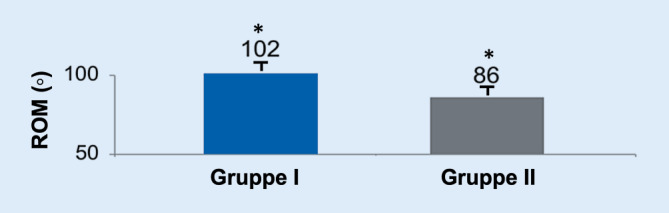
Um die Anzahl an Osteophyten zu quantifizieren, wurden CT-Bilder ausgewertet (Abb. 6). Ein Patient aus Gruppe I und 9 Patienten aus Gruppe II zeigten dabei Osteophyten von mehr als 1 mm Größe (*p* < 0,05).
